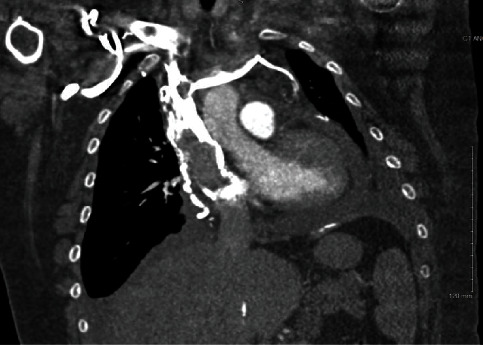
CT of the chest with SVC and subclavian thrombus.
